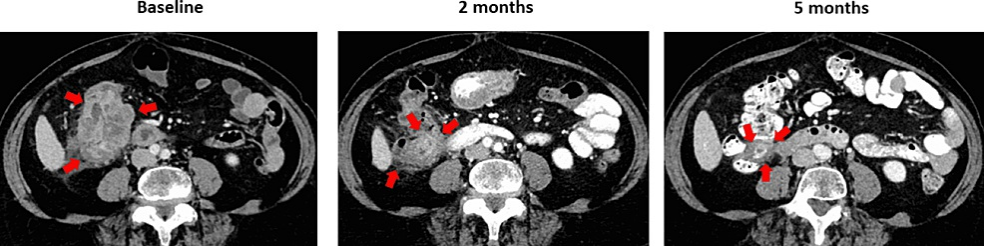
Serial CT scans at baseline, two months, and five months after immunotherapy initiation with ipilimumab and nivolumab combination demonstrating progressive shrinkage of the right hepatic flexure mass (indicated by red arrows).
